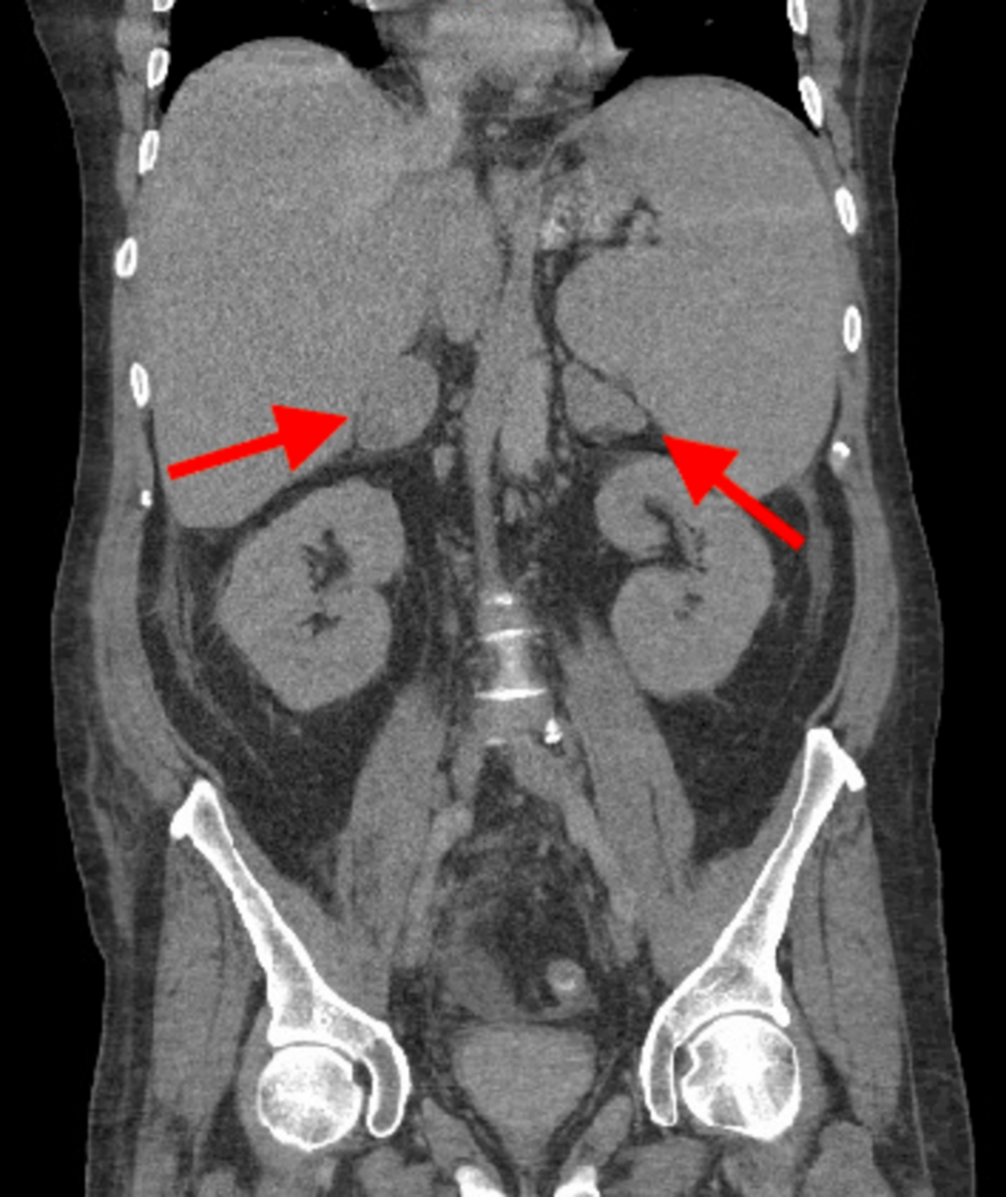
CT of the abdomen and pelvis showing bilateral adrenal masses
